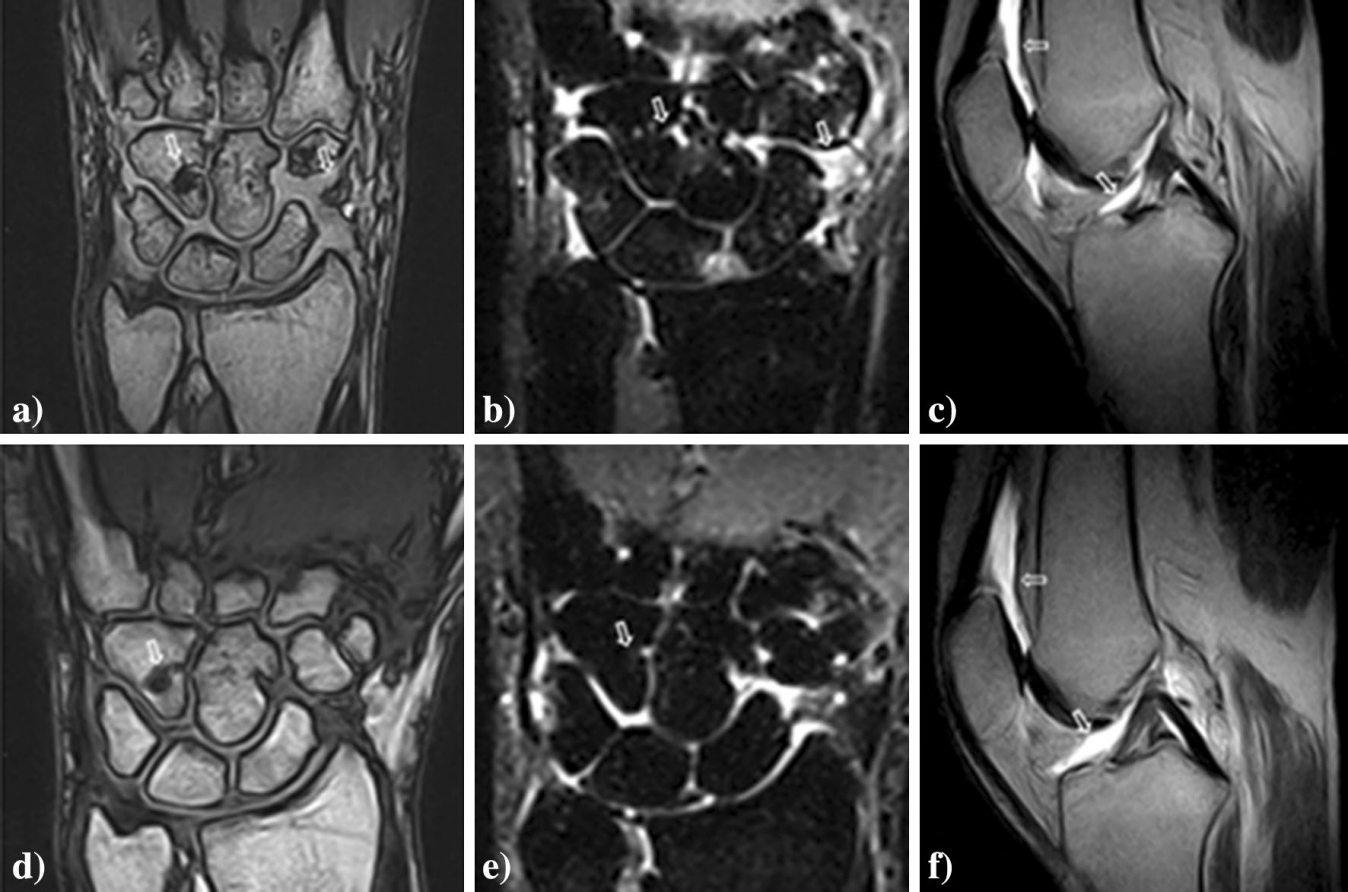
MRI findings before and after therapies in AOSD. In panels (a) and (b), T1-weighted and T2-STIR images of coronal sections on wrist are shown, respectively, before therapies (a,b), whereas, in panels (d) and (e), T1-weighted and T2-STIR images of coronal sections on wrist are shown, after therapies, reporting the reduction of synovitis, the disappearance of bone oedema, and the stabilisation of bone erosions (d,e) (arrows). In panels (c) and (f), T2-weighted images of sagittal sections on knee are shown before (c) and after therapies (f), respectively, detailing a worsening of proliferative synovitis and effusion in suprapatellar bursa and intra-articular space (arrows).
